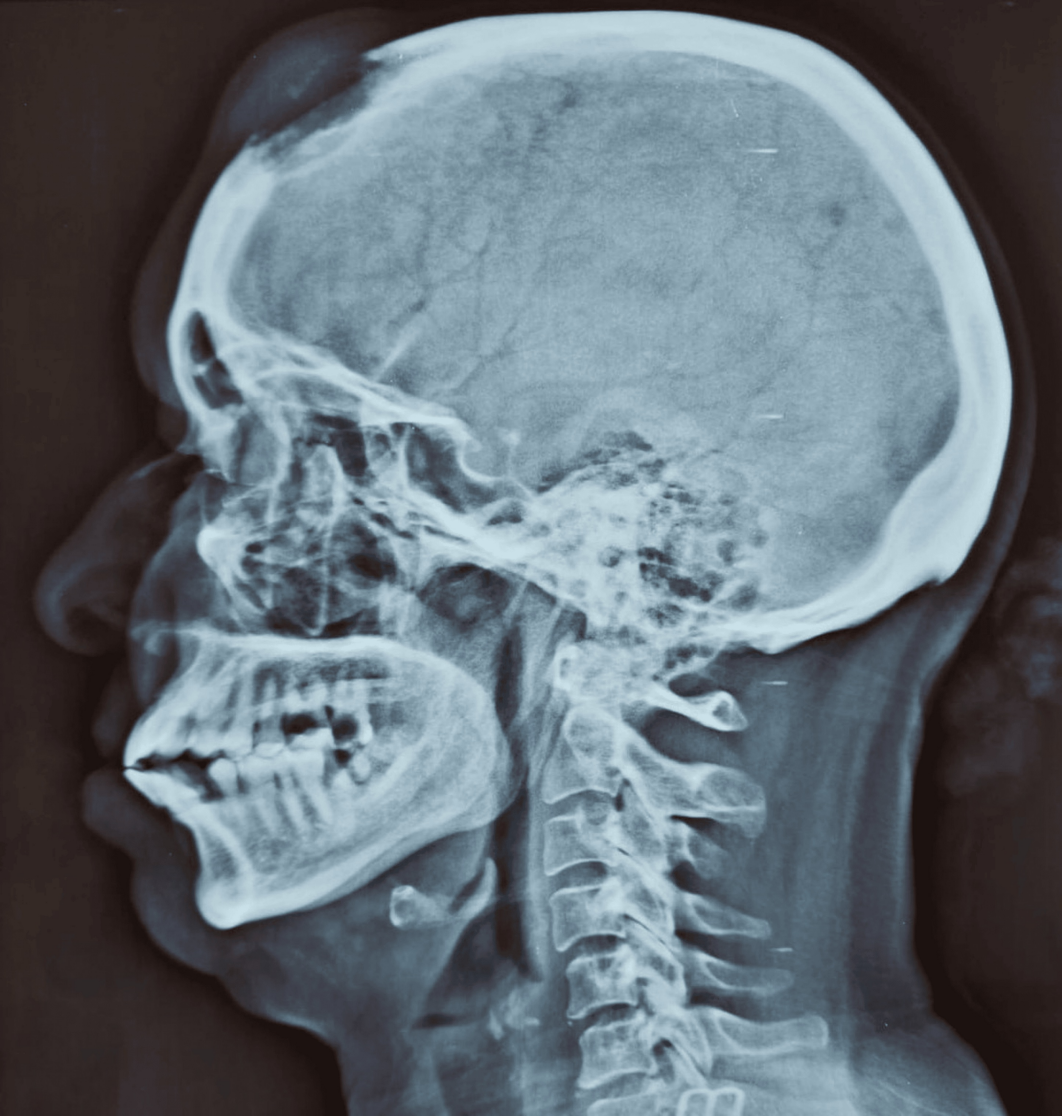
Lateral skull radiograph demonstrating a lytic lesion involving the median frontal bone with extension toward the subcutaneous scalp tissue.
